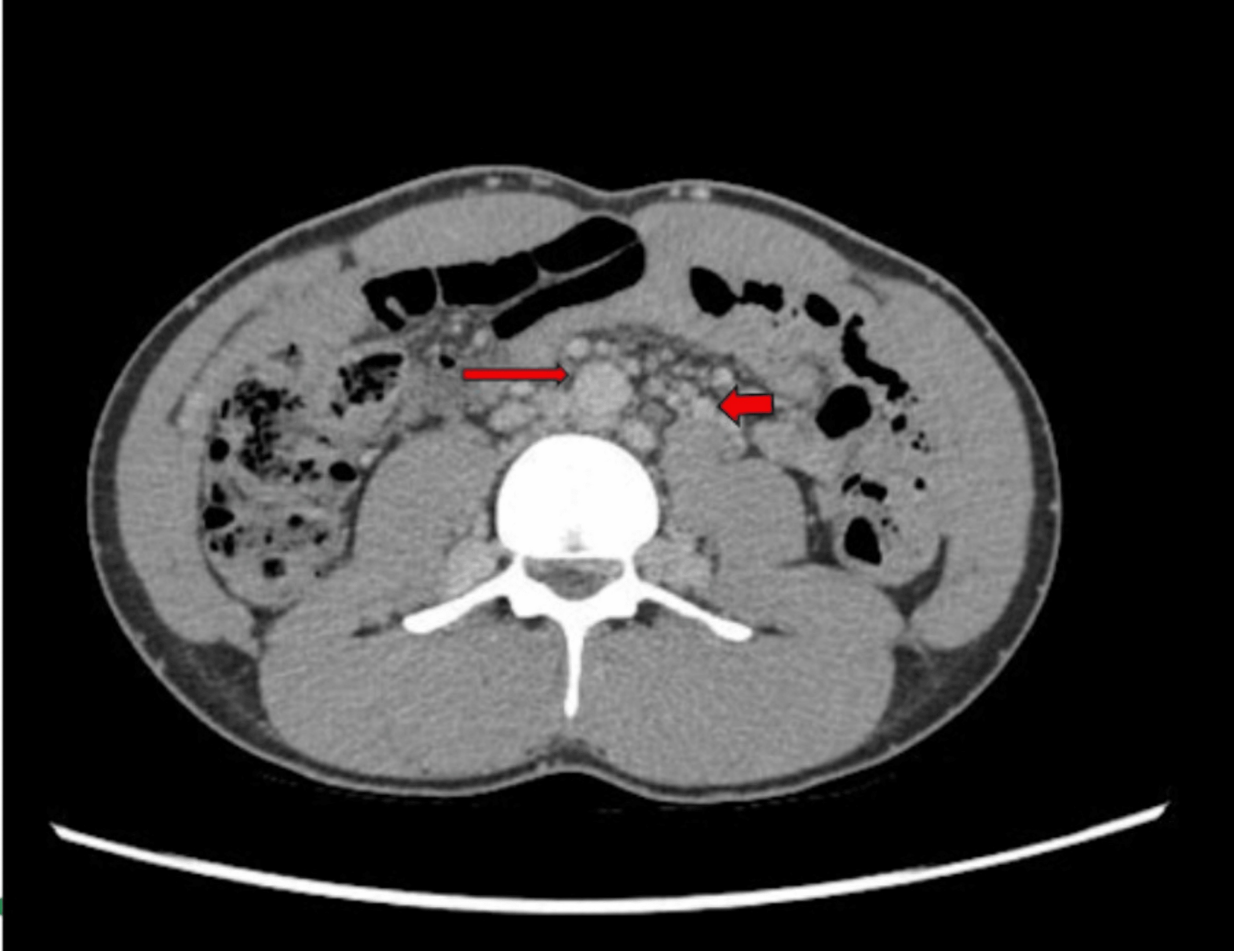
CT scan demonstrating absence of the IVC with extensive collateral venous development as a compensatory mechanism, consistent with IVC agenesis. IVC, inferior vena cava
